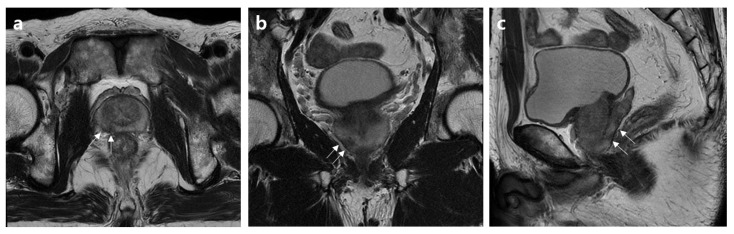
MR images of a 62-year-old patient without an extensive extraprostatic extension (EPE). (a) Axial, (b) coronal, and (c) sagittal T2-weighted MR images show indirect curvilinear contact length associated with a right peripheral zone mid-posterior lesion (arrows). The basic clinical findings included a serum PSA level of 16.5 ng/mL, a prostate volume of 36 mL, a positive biopsy core of 36%, clinical stage of T2c, biopsy grade 3, and intermediate D’Amico risk. The PI-RADS v2.1 scores and Mehralivand EPE grades on multiparametric MRI were 5 and 1, respectively, according to both radiologists.
